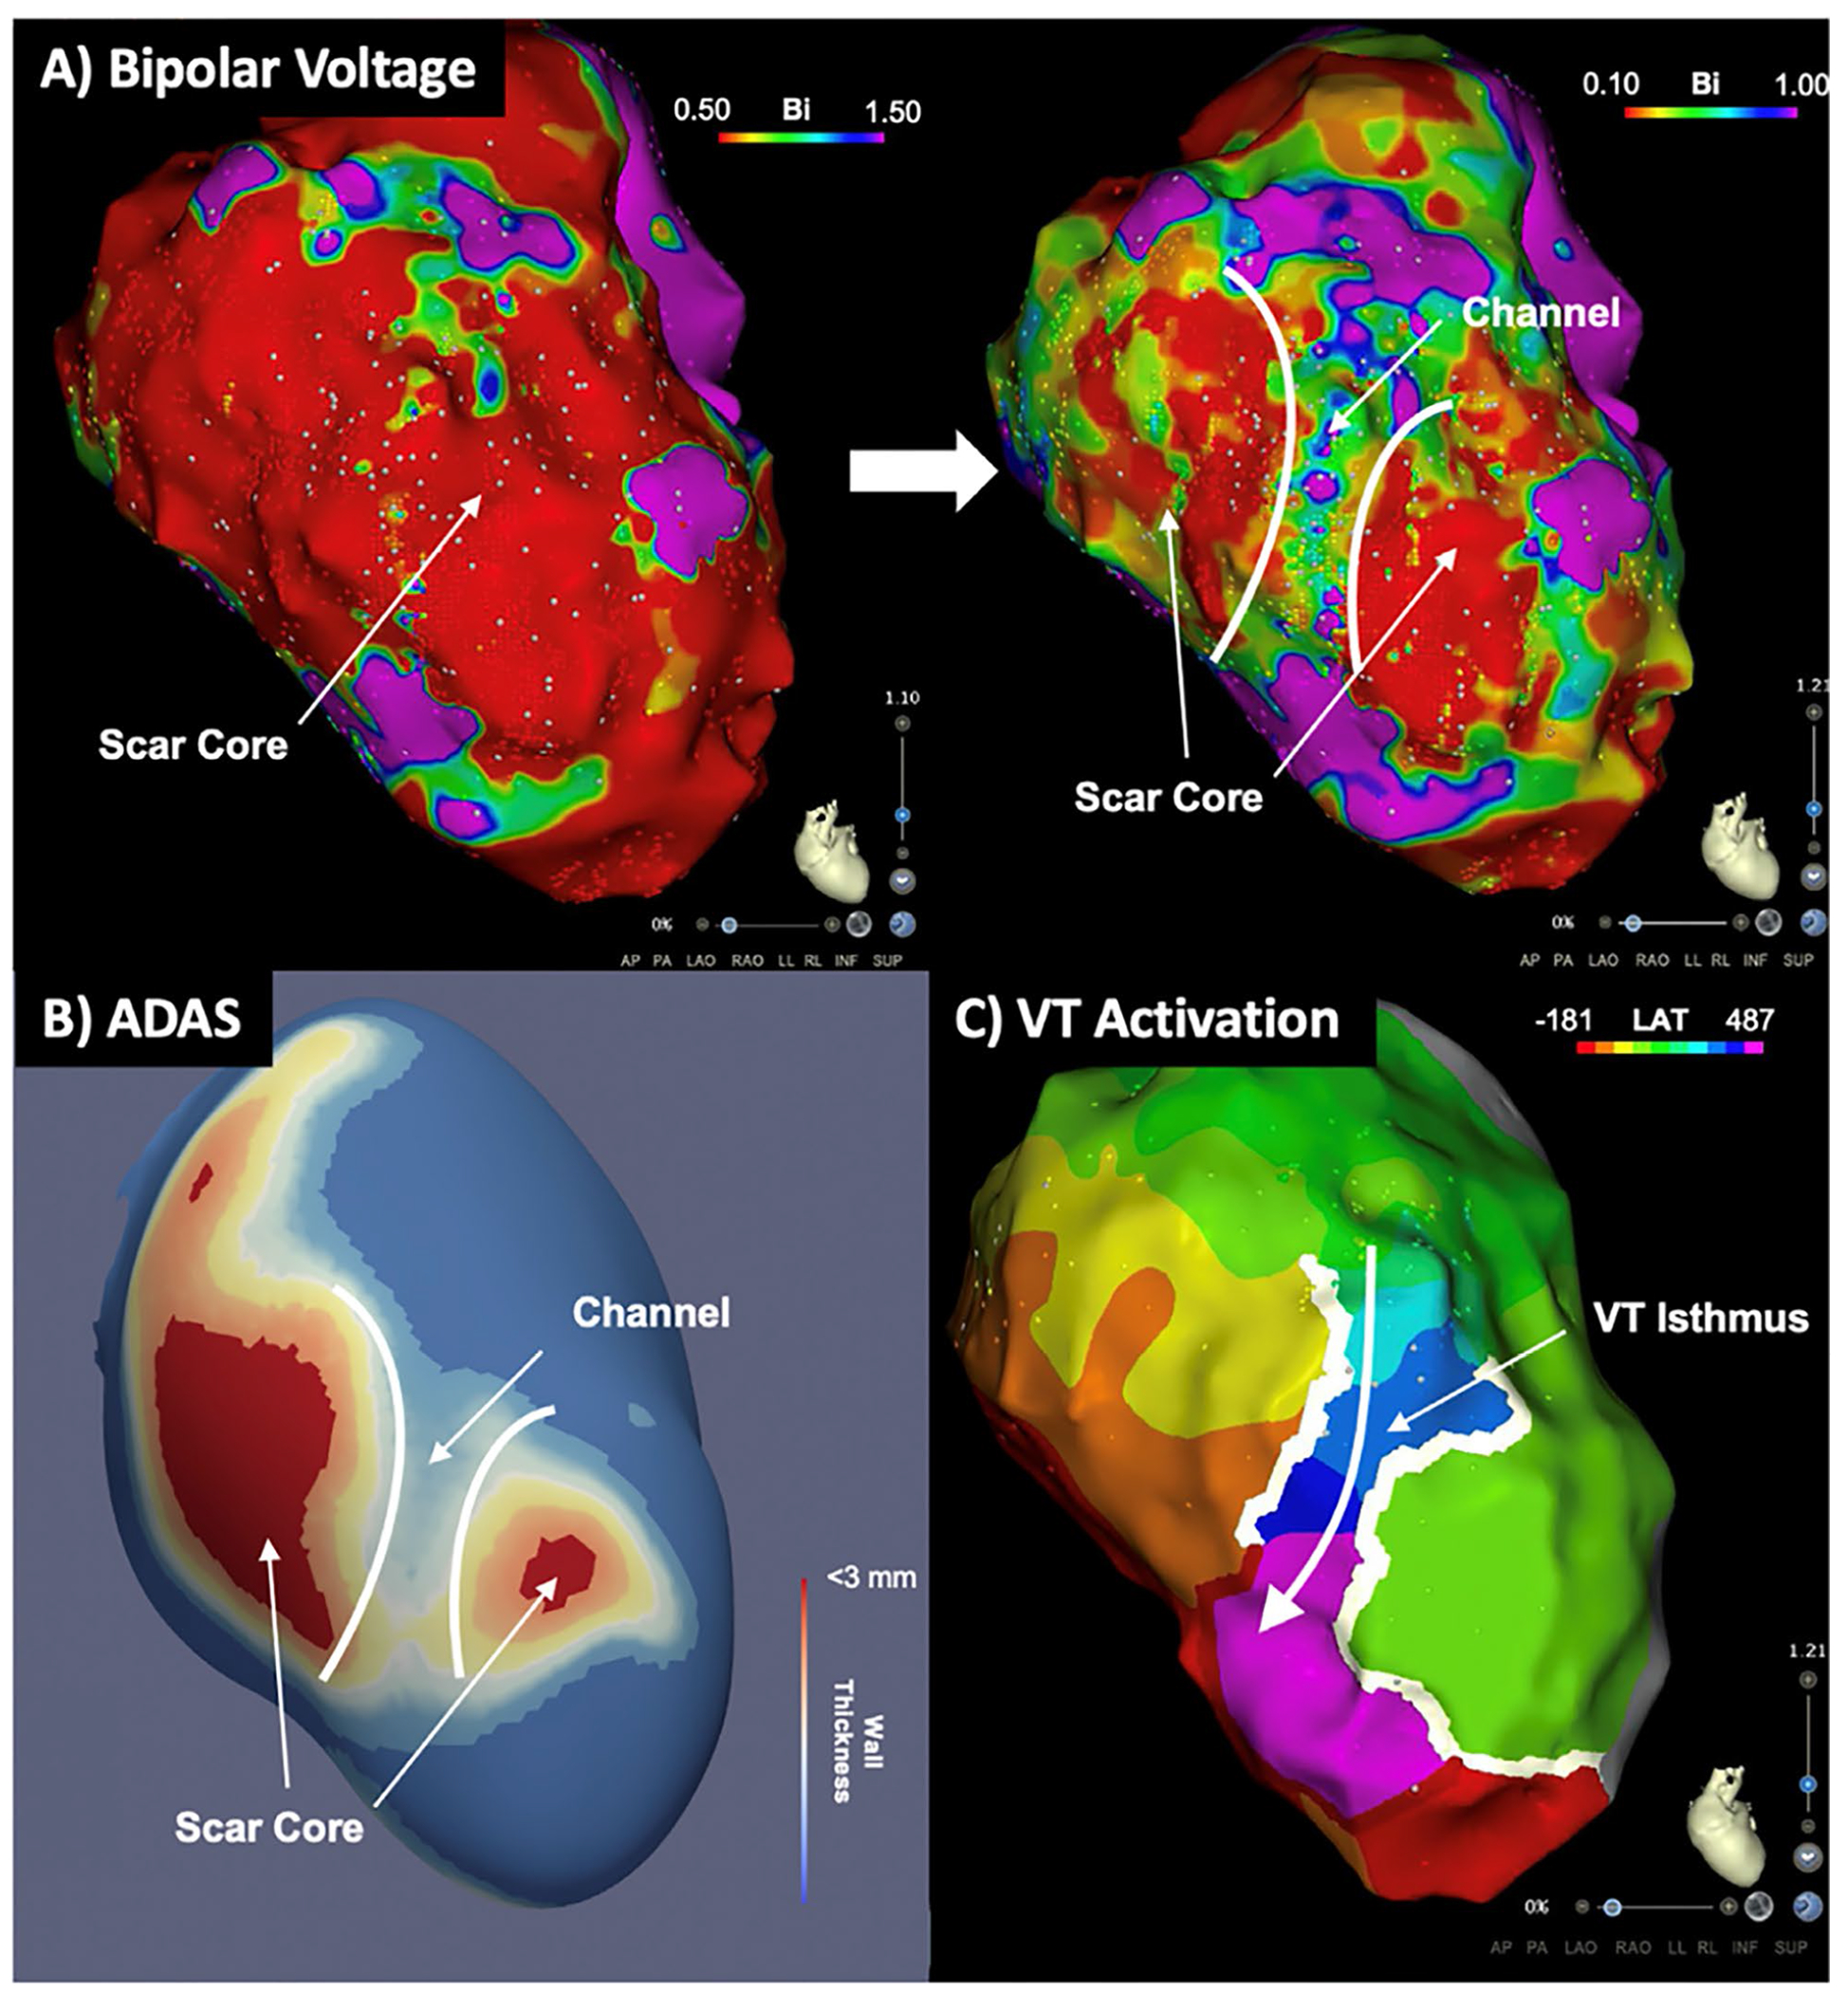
Voltage mapping with and without ADAS in a 72-year-old male with previous anteroseptal infarct: A voltage mapping using traditional range of 0.5–1.5 mV shows a large area of scar core over the antero-septum with no definite channel. Adjusting the voltage window to 0.1–1.0 mV reveals a channel between two areas of dense scar. B Pre-procedural cardiac CT with automated detection of arrhythmogenic substrate (ADAS). C Activation map of clinical VT, demonstrating conduction through the conducting channel identified with both ADAS and voltage mapping. (Used with permission from Dr. Geoffrey Lee, from [31]).
